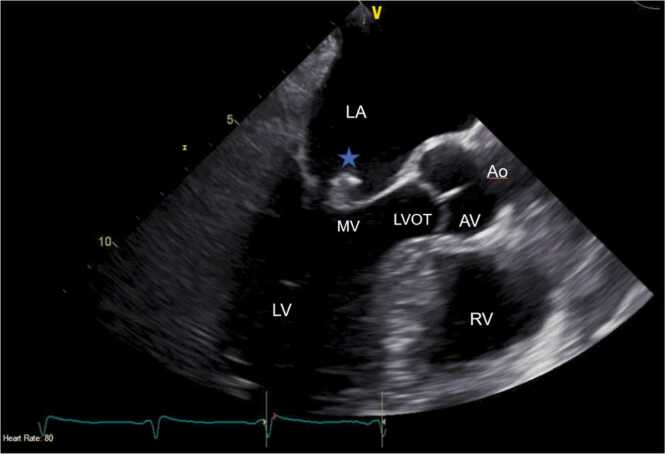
Transesophageal (TEE) ME LAX view revealing a large (1.73 × 0.55 cm), mobile vegetation (Blue star) on the atrial aspect of the anterior leaflet of mitral valve. LA = Left Atrium, LV = Left Ventricle, MV = Mitral Valve, RV = Right Ventricle, AV = Aortic Valve, LVOT = Left Ventricle Outflow Tract, Ao = Aorta.
